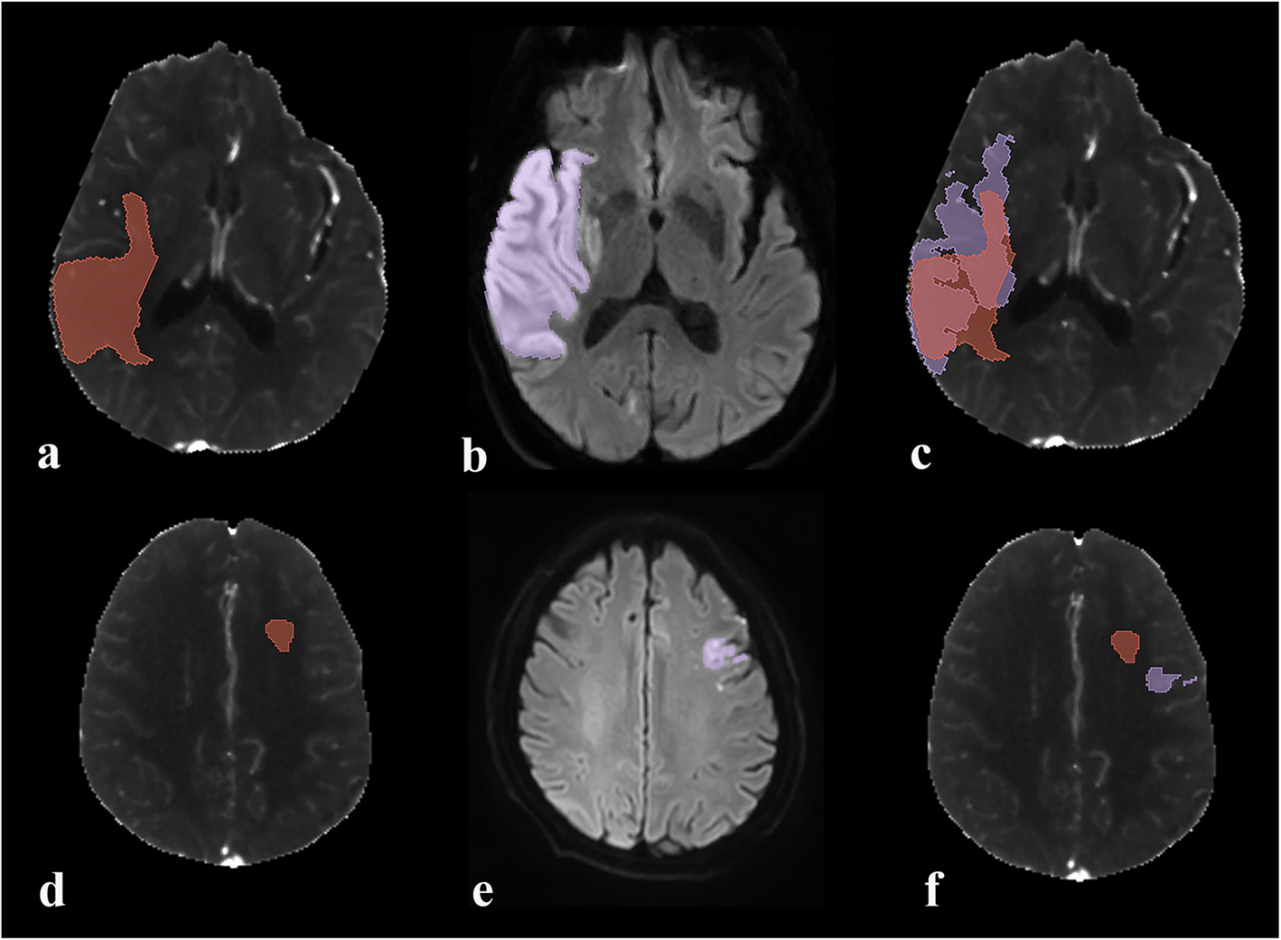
Assessment of spatiall agreement in two representative patients. a - c One 77-year-old male sufferred from the occlusion of right middle cerebral artery. The time interval from symptom onset to CTP scan was 324 minutes, and the time interval from CTP to recanalization was50 minutes. The volume of ischemic core on baseline CTP and infarct area on follow-up DWI was 81.1 mL and 118.7 mL, respectively. After the ischemic core was identified on CTP- derived CBF maps a and infart area was manually outlined on post-treatment DWI b, infarct area on post-treatment DWI was registered to CTP c. The Dice similarity coefficient was 0.70, and the average Hausdorff distance was 0.35 mm. d - f One 47-year-old male sufferred from the occlusion of left internal carotid artery. The time interval from symptom onset to CTP scan was 199 minutes, and the time interval from CTP to recanalization was 85 minutes. The volume of ischemic core on baseline CTP was 2.60 mL, and the volume of infarct area on follow-up DWI was 2.23 mL. After the ischemic core was identified on CTP- derived CBF maps d and infart area was manually outlined on post-treatment DWI e, infarct area on post-treatment DWI was registered to CTP f. The Dice similarity coefficient was less than 0.01, and the average Hausdorff distance was 16.85 mm
